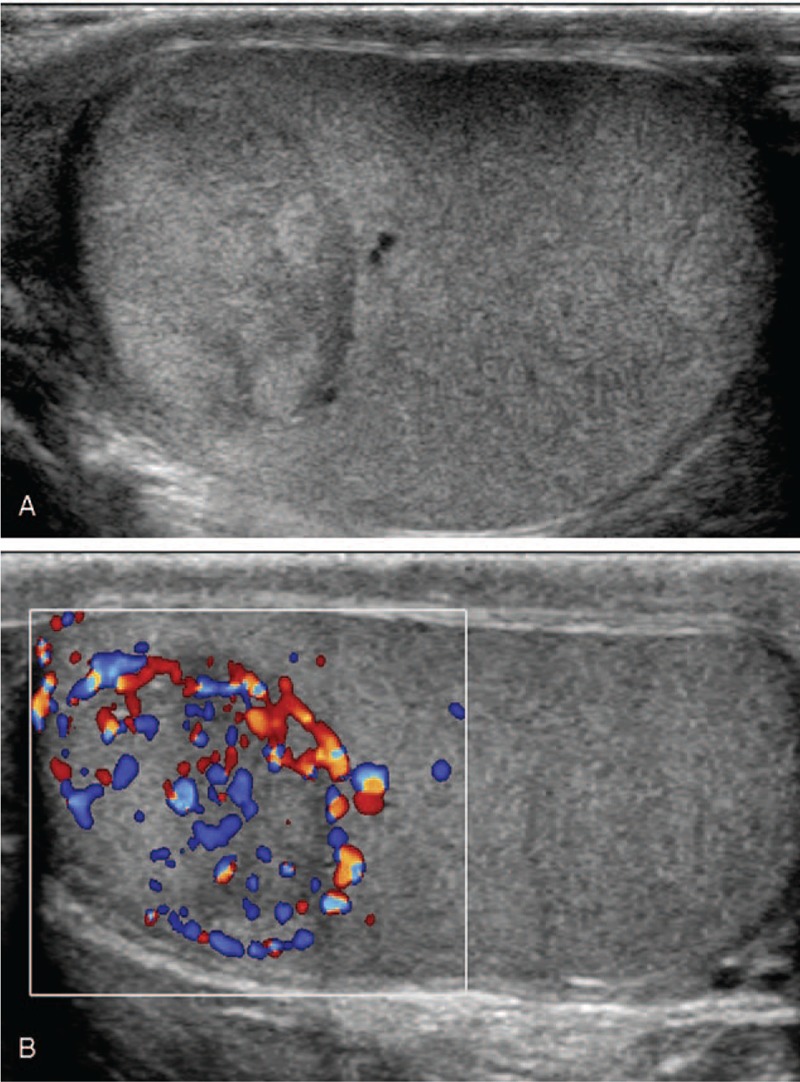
(A) Longitudinal image of the testis demonstrating a well-circumscribed, hypoechoic solid mass in the upper part of the testis. (B) Longitudinal color Doppler image of the testis demonstrating abundant blood flow signals in the mass.
